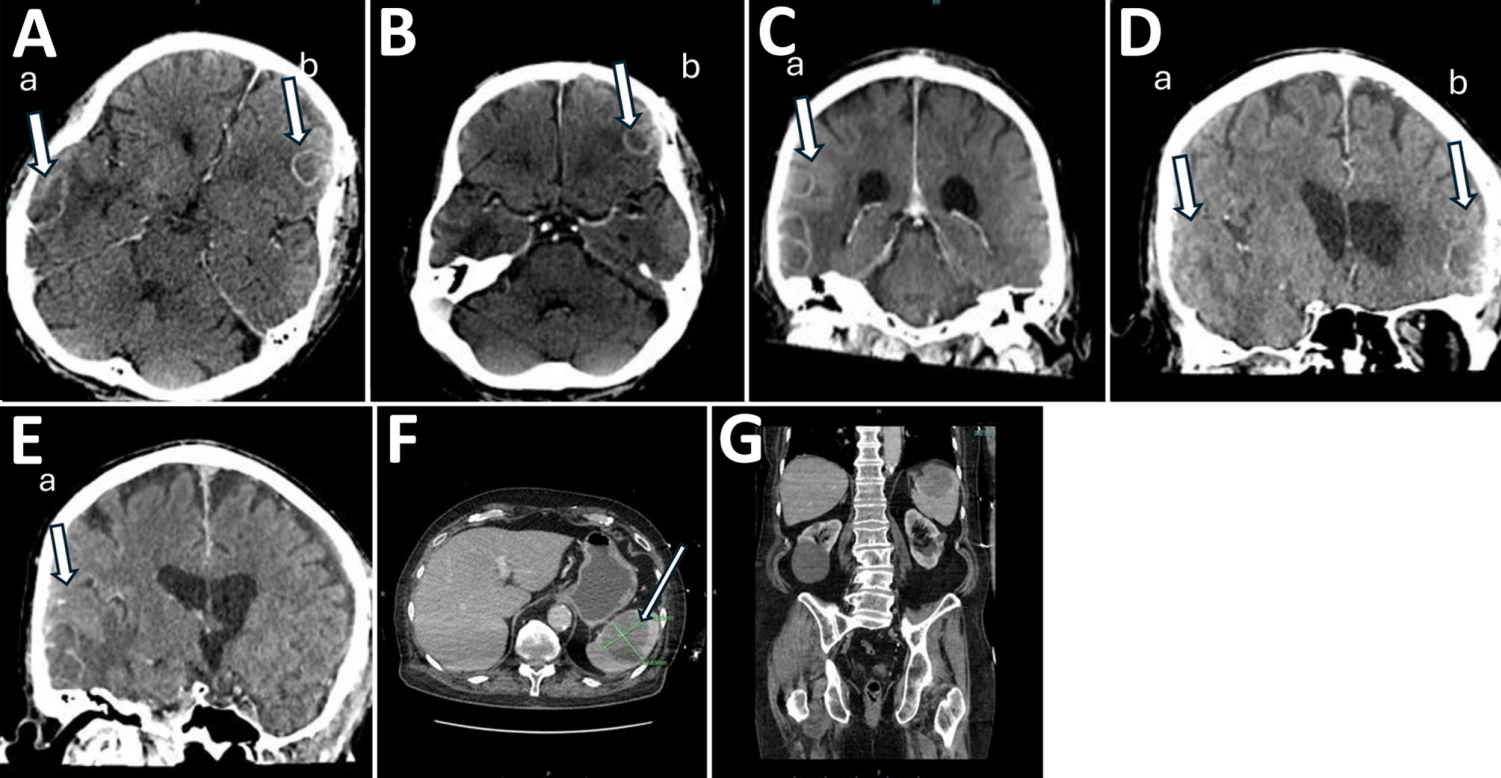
Computed tomography (CT) images of brain, abdomen, and spine of 79-year-old man with chronic obstructive pulmonary disease and large B-cell lymphoma who had disseminated *Nocardia ignorata* infection, United States. A–E) Brain CT with and without contrast showing multiple regular peripheral enhancing brain lesions (indicated by arrow and lowercase letters a and b) measuring 2.2 cm at the left frontal lobe (A) and 3.8 cm at the right (B); mildly lobulated at the right posterior lateral temporal lobe (C); and moderate edematous mass (D), resulting in mild brain herniation (E). F, G) CT images of abdomen (F) and pelvis (G). An indeterminate complex lesion measuring 6.1 cm is seen in the spleen (F, arrow).
